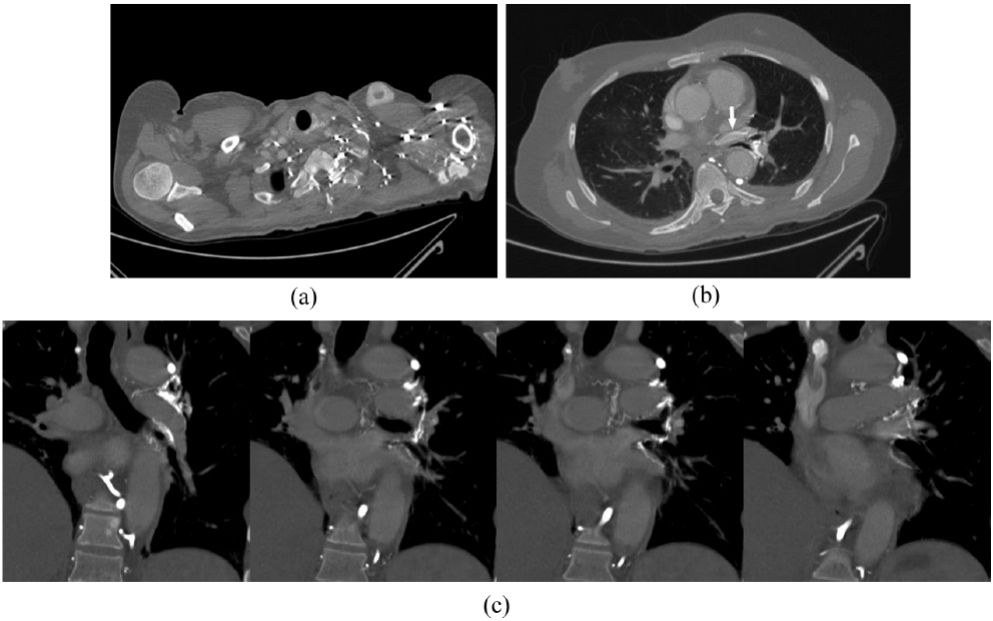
Chest computed tomography angiogram conducted under pulmonary embolism protocol. Contrast was injected into a left upper extremity vein. (a) Venous collaterals are well-developed as a result of left brachiocephalic vein stenosis. (b) Contrast is seen within the left superior pulmonary vein (arrow). (c) Four coronal slices are shown, demonstrating collateralization of the left bronchial venous plexus as well as enhancement of the left pulmonary vein.
